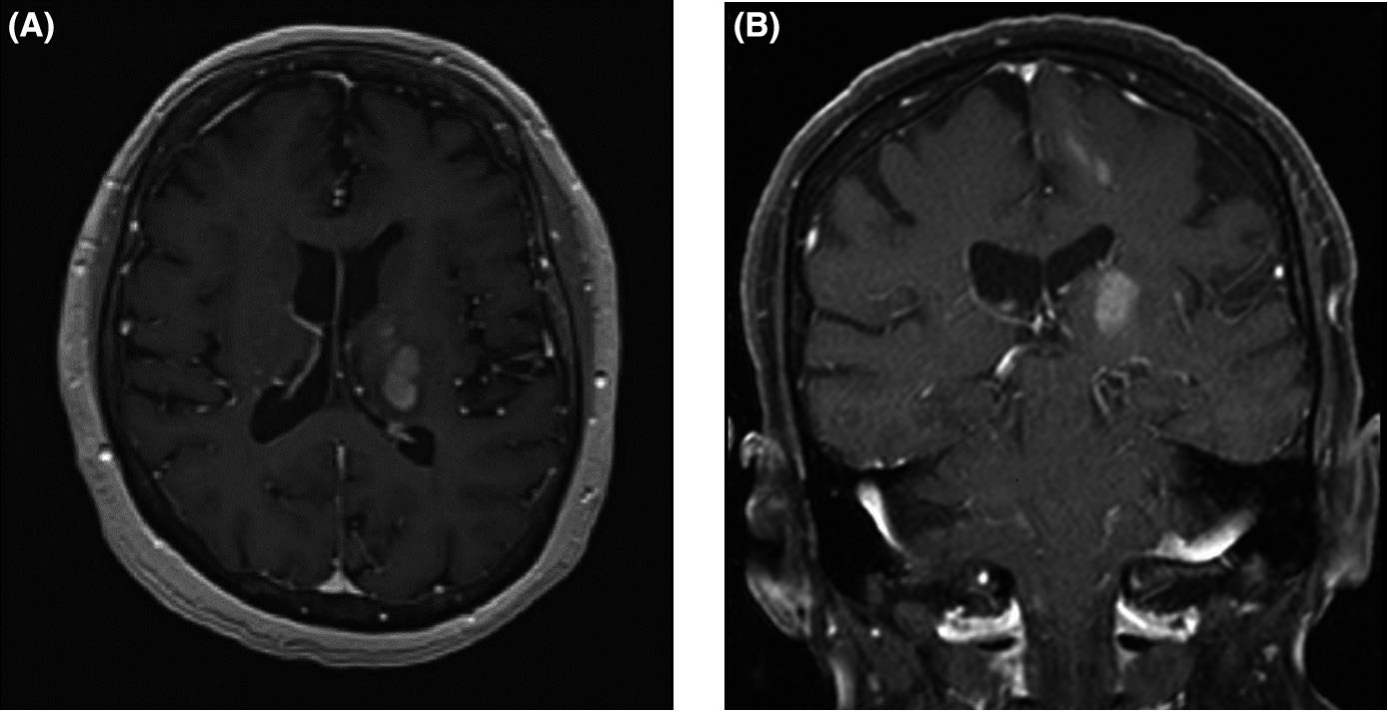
T1‐weighted post‐contrast magnetic resonance imaging (MRI) of the brain obtained when the patient was initially diagnosed with Bing‐Neel Syndrome. An expansile mass with associated heterogeneous internal enhancement is observed within the left thalamus measuring 3.3 × 2.5 cm
